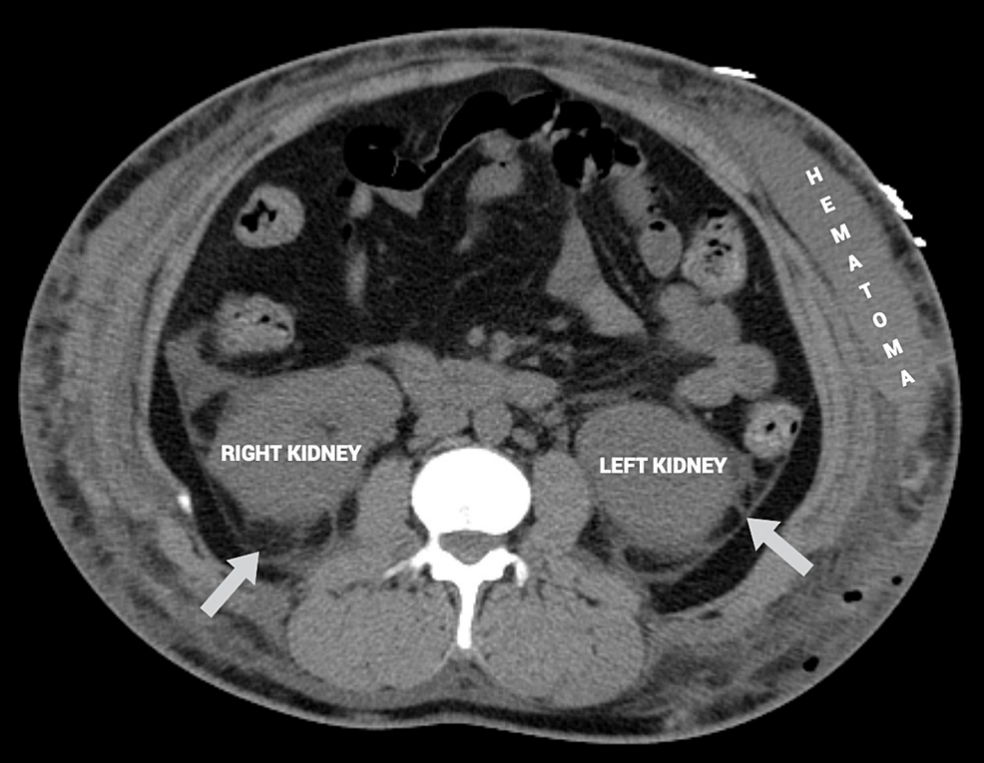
Computed tomography axial view. Perinephric fat stranding around both kidneys is shown (arrows). Hyperdense hematoma fluid collection on left side is shown. Perinephric - adjacent to the kidney
